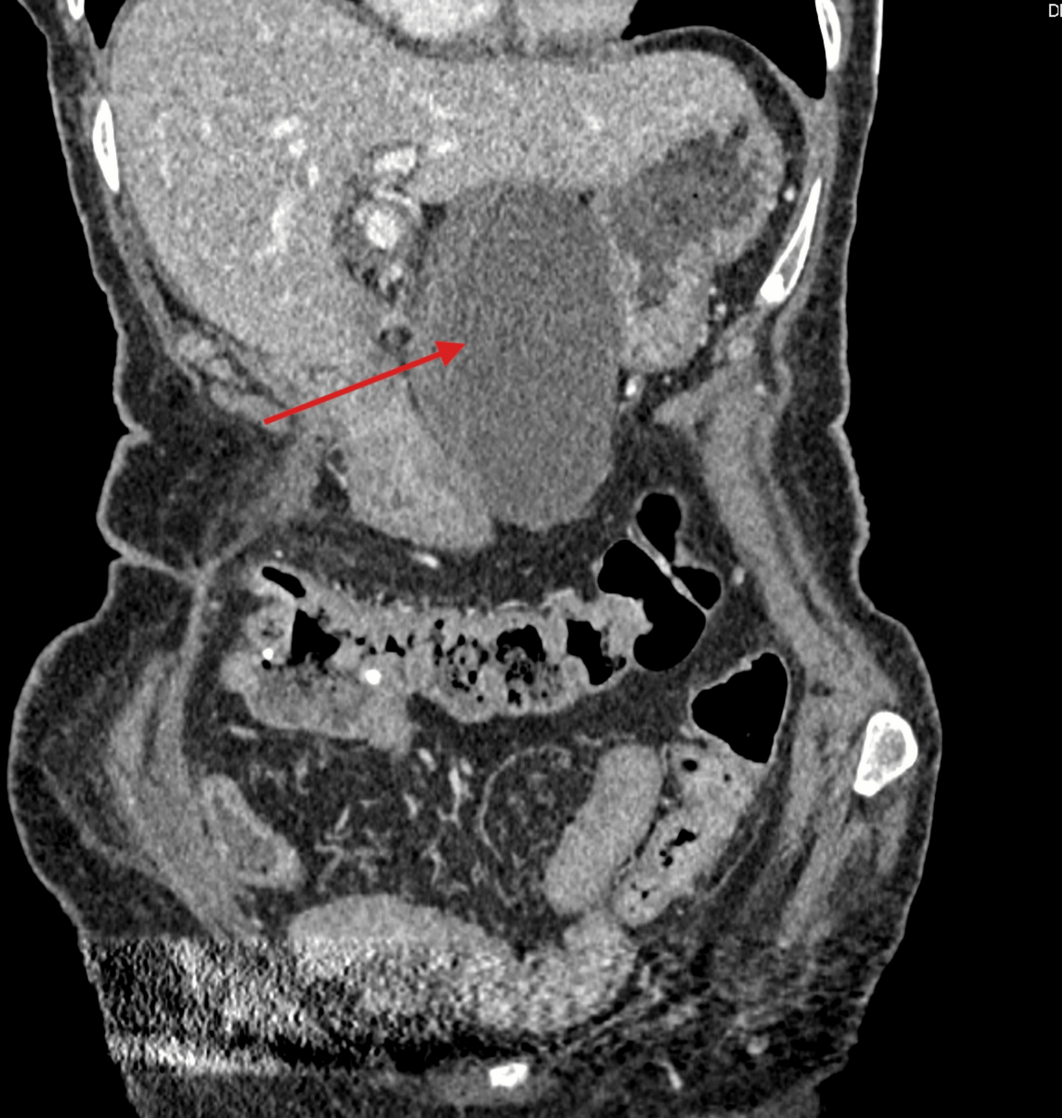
CT scan of the abdomen and pelvis showing a grossly distended gallbladder abnormally positioned in the midline (red arrow).
